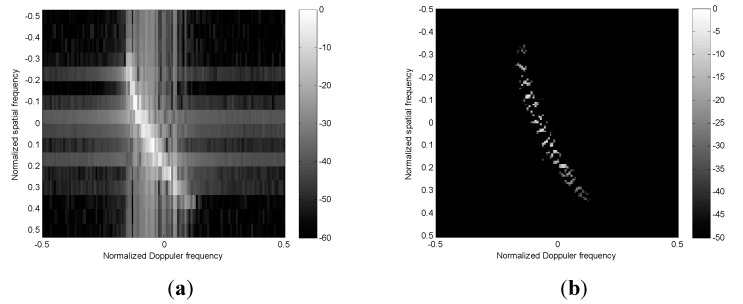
The clutter angle-Doppler spectrum image with a 10.5 km slant distance. (a) 2D FFT; (b) Spatial RDSR.
